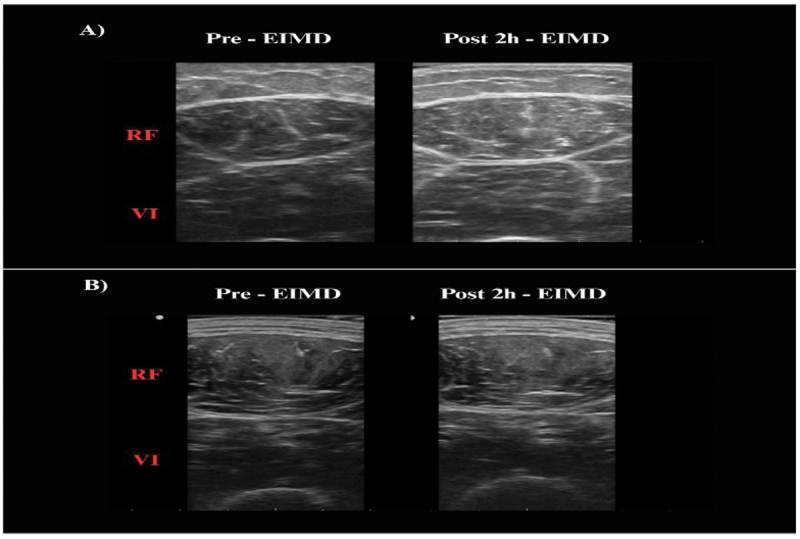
Representative ultrasound images and echo intensity measurements. Images were taken before and 2 hours after EIMD for the PLA group (A) and the EPP-AF group (B). RF = rectus femoris muscle; VI = vastus intermedius muscle.
